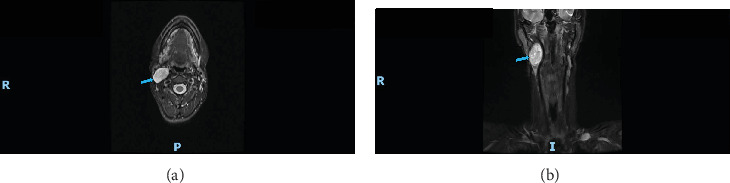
Angiographic MRI. (A) Axial view and (B) coronal view.
